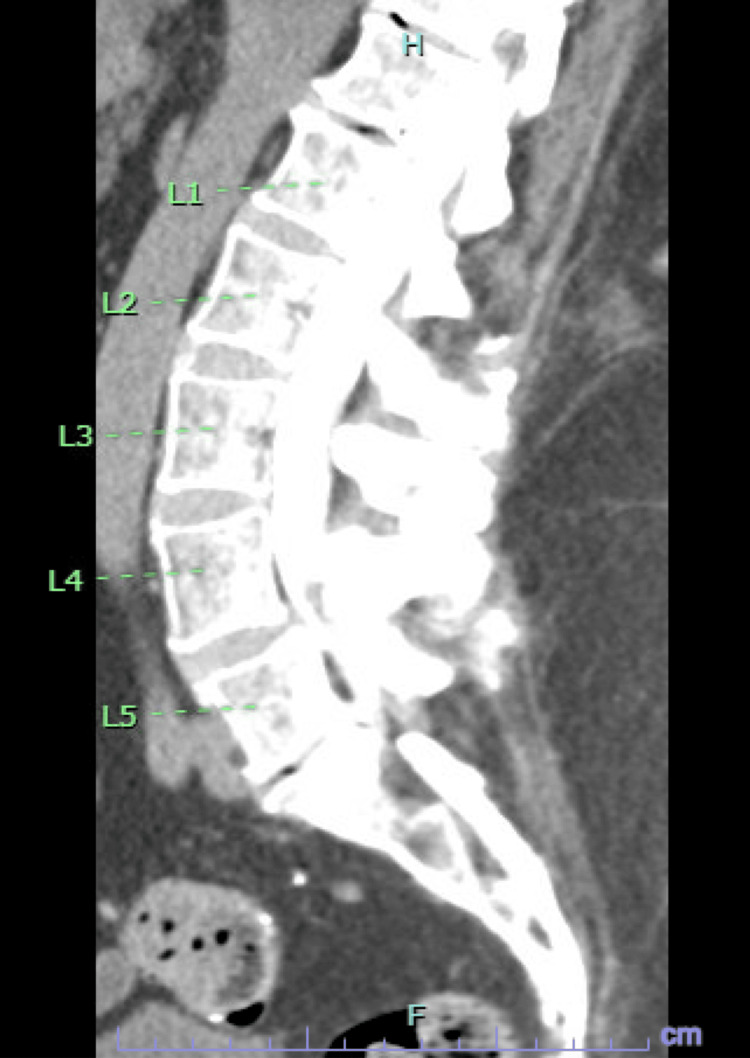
Sagittal lumbar spine CT with contrast Multifactorial moderate to severe central canal stenosis at L4-L5. Negative for neural foraminal stenosis. Degenerative disc disease with vacuum phenomenon T12-L1 and L5-S1. Negative for focal disc herniation, central canal or neural foraminal stenosis.
